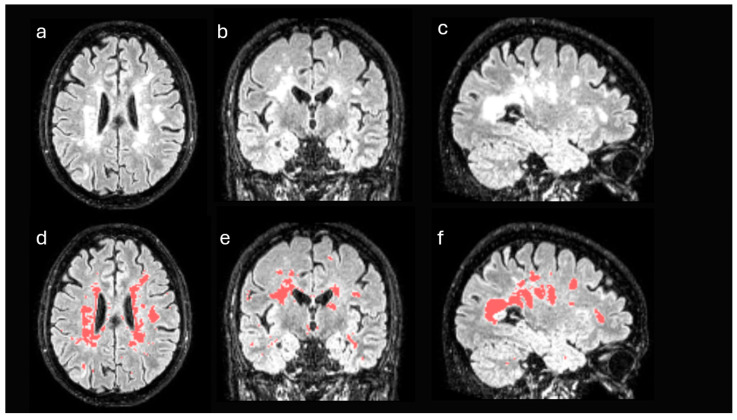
Damaged white matter segmentation on FLAIR-MRI. Axial (a), coronal (b), and sagittal (c) views. Lesions were identified using a semi-automated thresholding method. Damaged areas are highlighted in red in (d–f).
